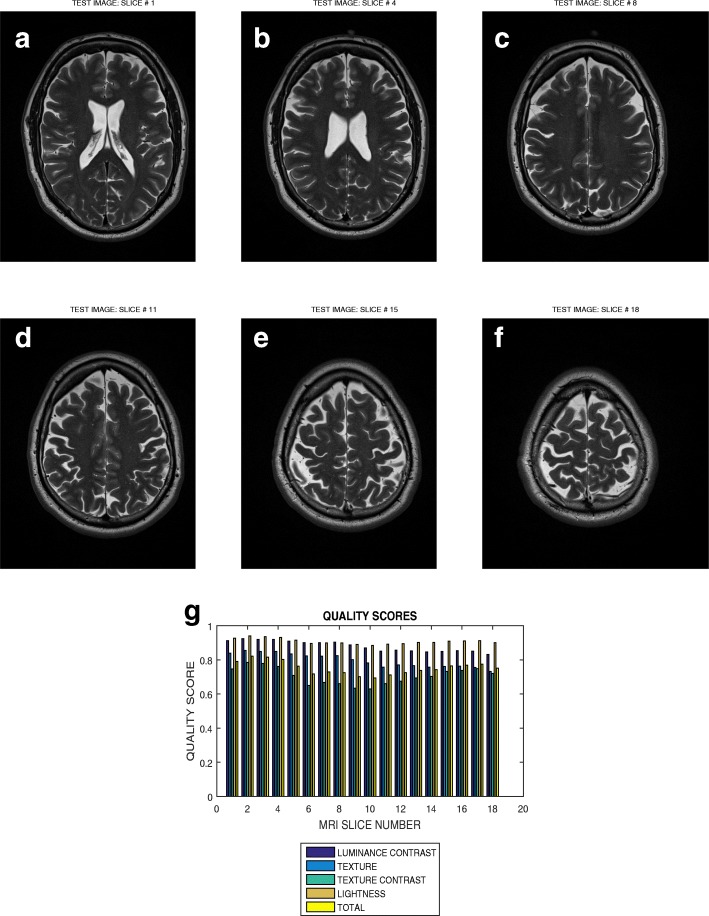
Six slices with indices (a) 1, (b) 4, (c) 8, (d) 11, (e) 15 and (f) 18 in a T2 weighted MRI volume data from BrainCare Oy, (g) luminance contrast, texture, texture contrast, lightness and total quality scores of 18 successive slices in the MRI volume data
